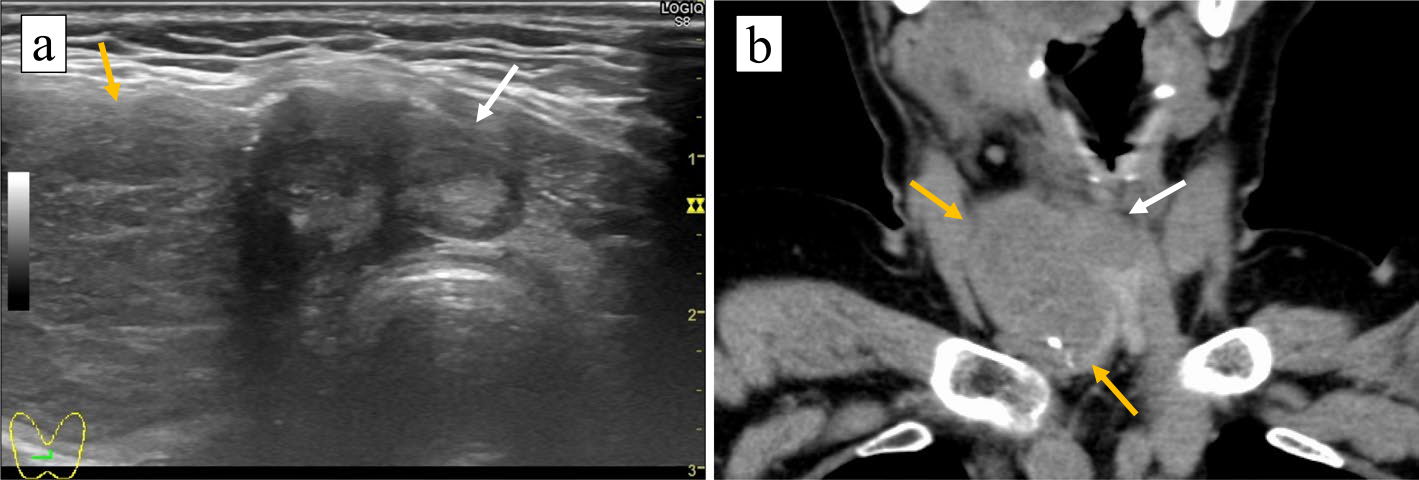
a Representative imager of ultrasonography. A well-defined mass lesion in the right lobe of the thyroid gland (yellow arrow) and an indistinct mass lesion in the isthmus (white arrow) are demonstrated. b Representative image of CT scan. The tumor of right lobe of the thyroid gland (yellow arrow) and mass lesion in the isthmus (white arrow) are demonstrated
